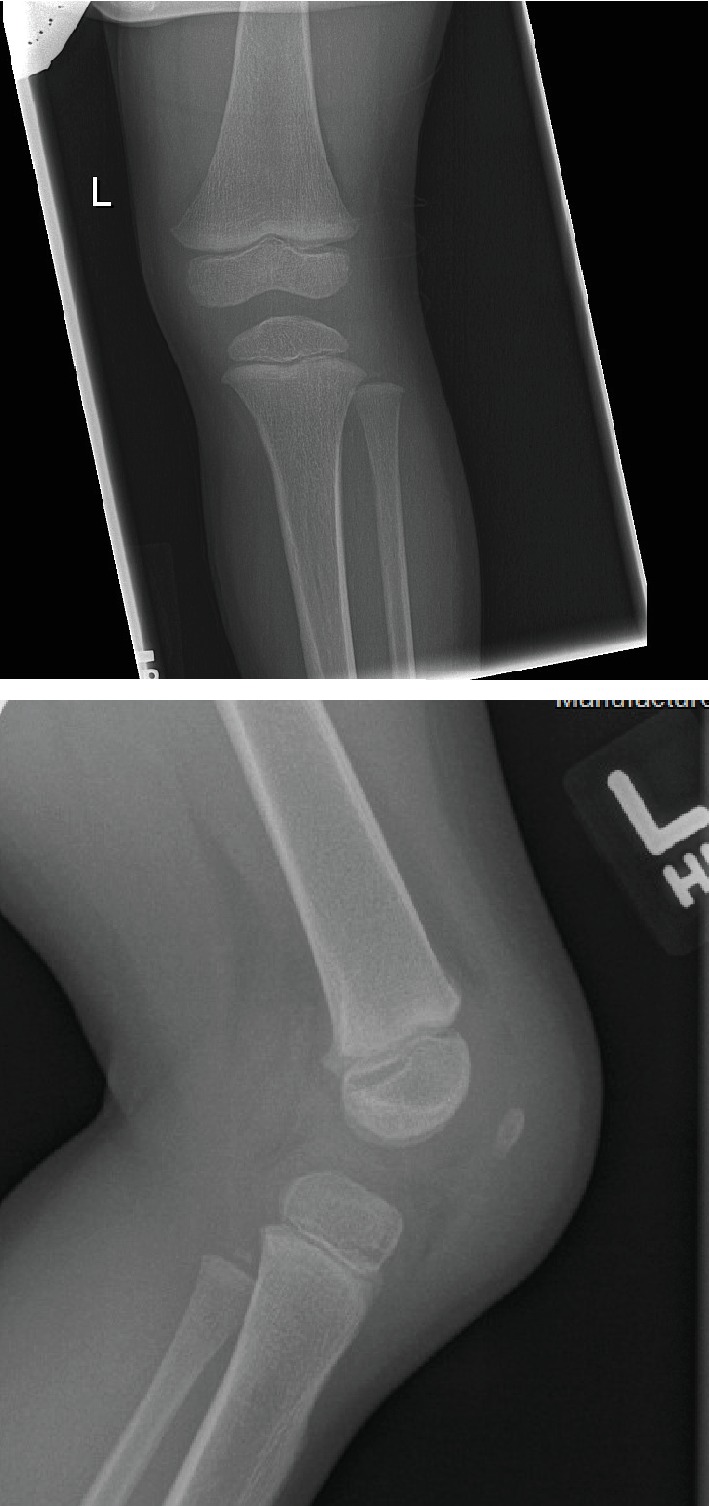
AP and lateral radiograph of the affected knee shows no obvious bony injury or effusion but notable prepatellar soft tissue swelling.
